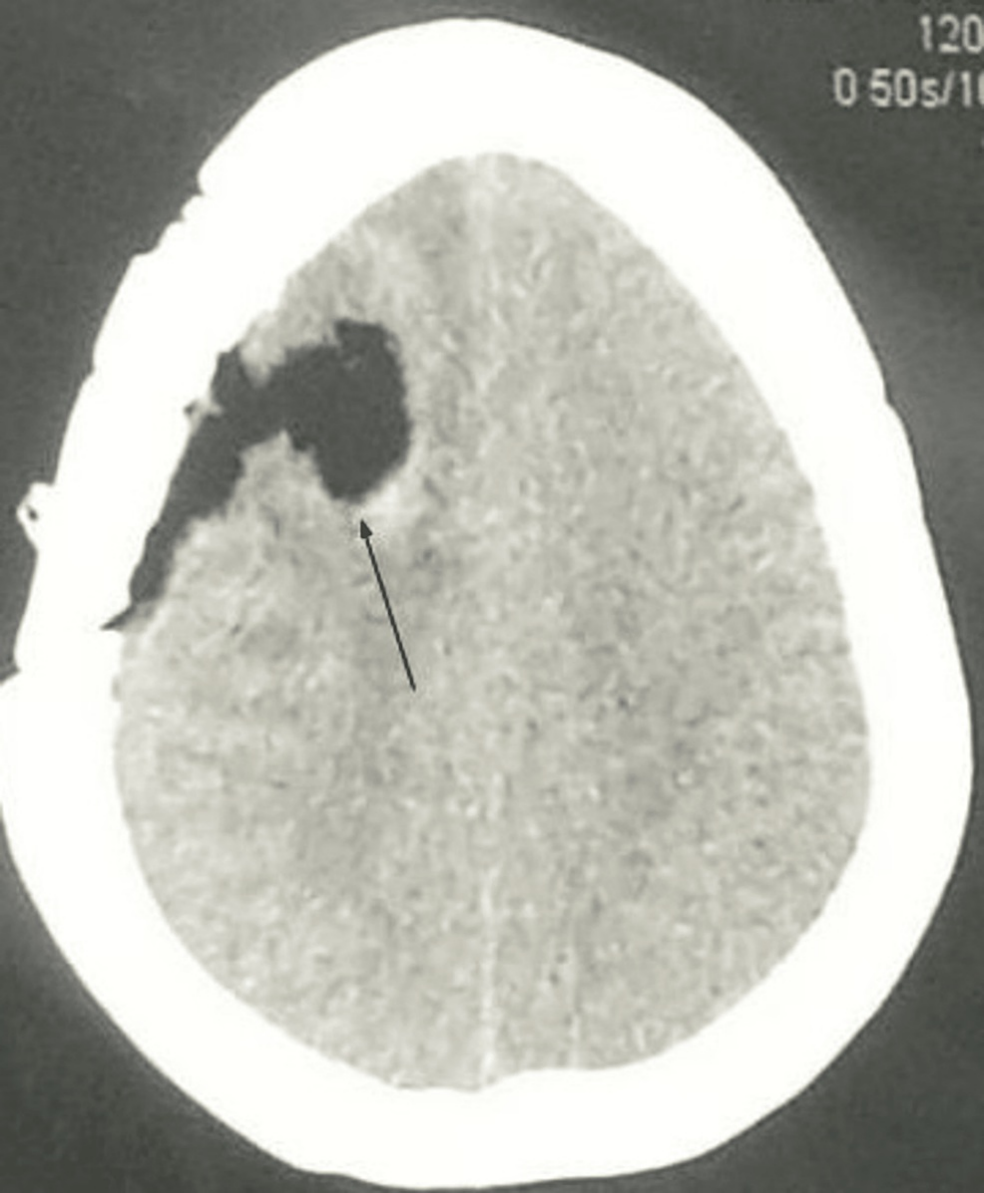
Post-operative CT scan shows excision of brain tumor (arrow)
